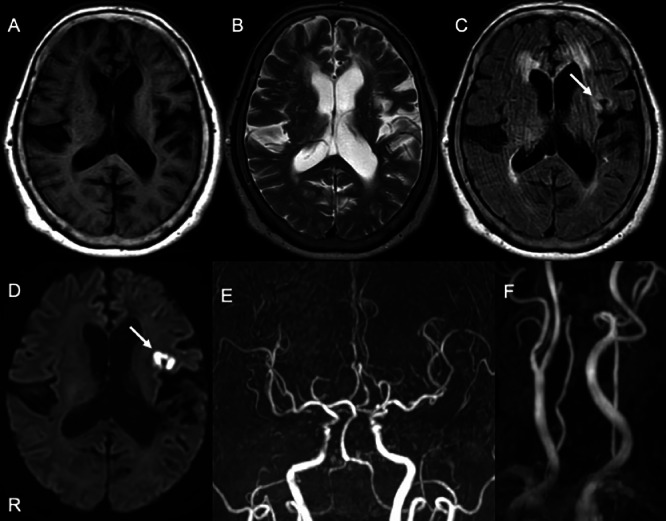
Magnetic resonance imaging (MRI) obtained at admission. (A) T1-weighted image, (B) T2-weighted image, (C) FLAIR image, (D) diffusion-weighted imaging (DWI), (E) intracranial magnetic resonance angiography (MRA), and (F) cervical MRA. DWI (D) demonstrates an acute ischemic infarction in the left frontal lobe (white arrow), which is also hyperintense on FLAIR (white arrow), indicating no DWI–FLAIR mismatch. (DWI was acquired using a single-shot echo-planar sequence; TR/TE = 4000/88 ms, b = 1000 s/mm²). FLAIR images show periventricular white matter hyperintensities and cerebral atrophy, more prominent in the left hemisphere. Both intracranial and cervical MRA reveal no significant stenosis or occlusion in the major cerebral or cervical arteries, and no lateralized vascular abnormalities. The letter ‘R’ indicates the right side of the patient in all images.
